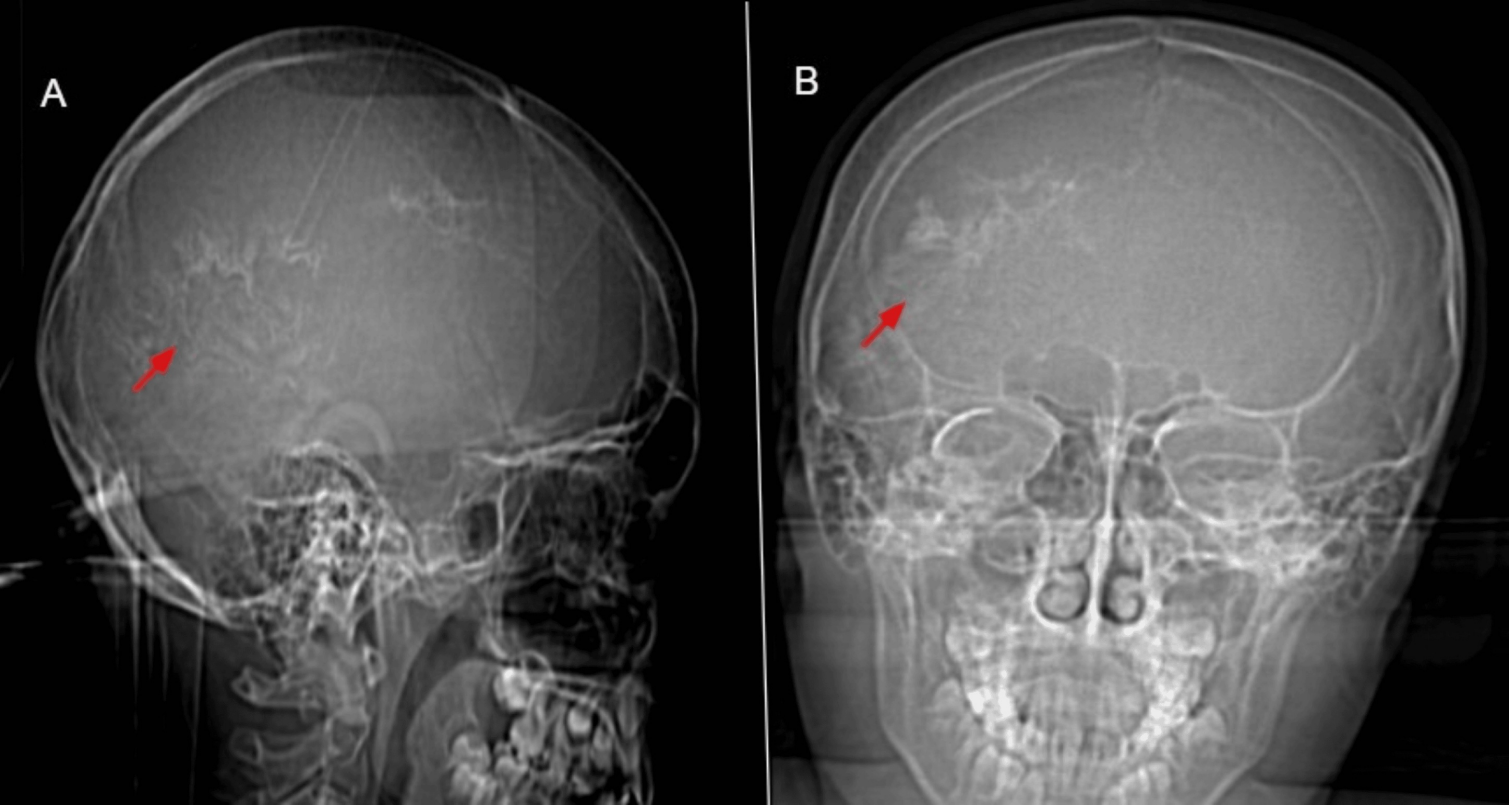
Lateral and frontal scout CT images at six years of age Lateral (A) and frontal (B) scout CT images at six years of age demonstrate right-sided gyriform ‘tram-track’ calcifications of the skull, corresponding to underlying parenchymal calcifications.
